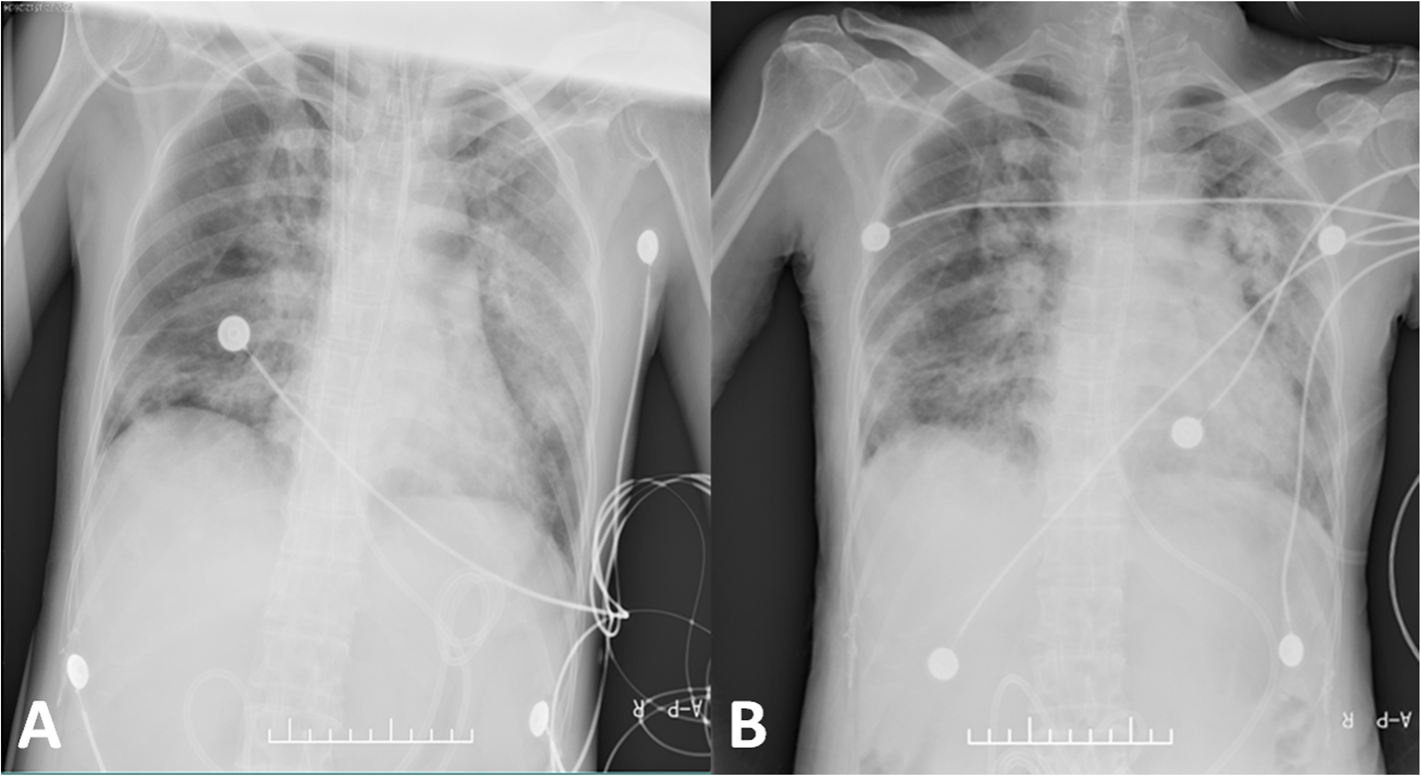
a Chest radiograph on the 19th day of admission. b Chest radiograph on the 35th day of admission
